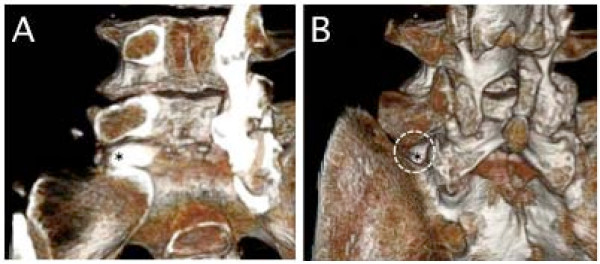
Case 13. A three-dimensional CT-discogram obtained from a 78-year-old male who presented with severe left leg pain. (A) The herniated disc (*) was located from the intraforaminal to the extraforaminal lesion. (B) A tubular retractor was set at the dotted area, and the herniated disc (*) was removed.
